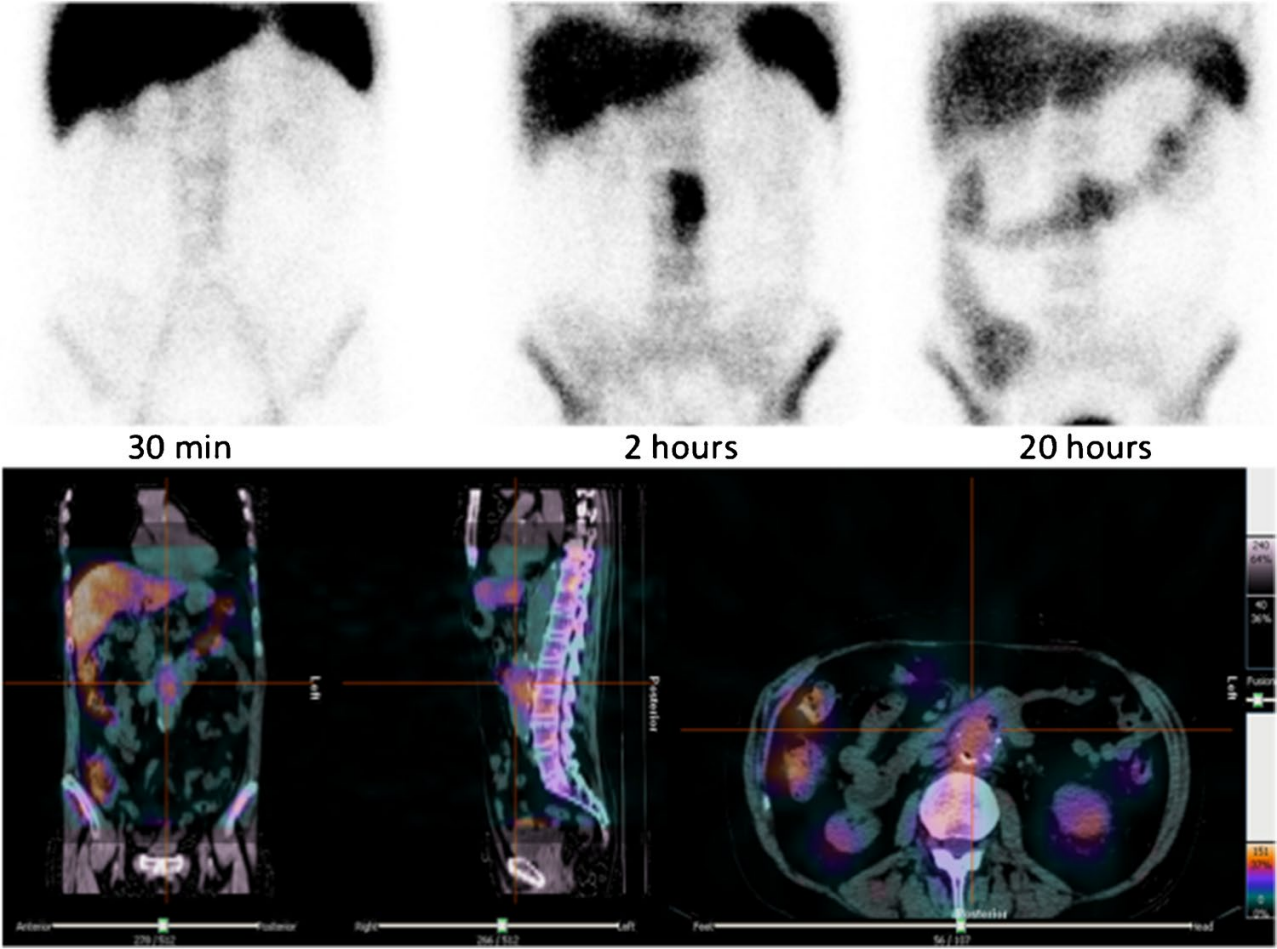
Planar images of WBC scintigraphy, acquired at 30 min, 2 and 20 h p.i., show an increased uptake over time, that is consistent with an infection of abdominal graft (upper panel). SPECT/CT images, acquired 2 h p.i., allow to accurately localize the uptake and to evaluate its extent (lower panel)
